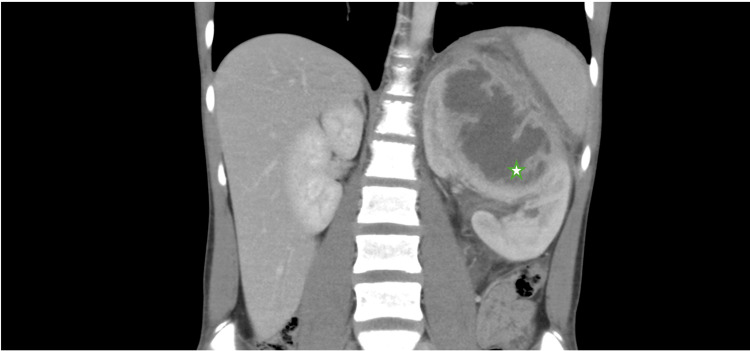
CT imaging (coronal view) Demonstrating a complex solid and cystic mass involving the upper to mid pole of the left kidney measuring 11.5 x 7.9 x 10 cm (star), with multiloculation, septations, perinephric micro-collections, extensive perinephric stranding and fluid, and enhancing urothelial thickening of the upper left renal collecting system.
